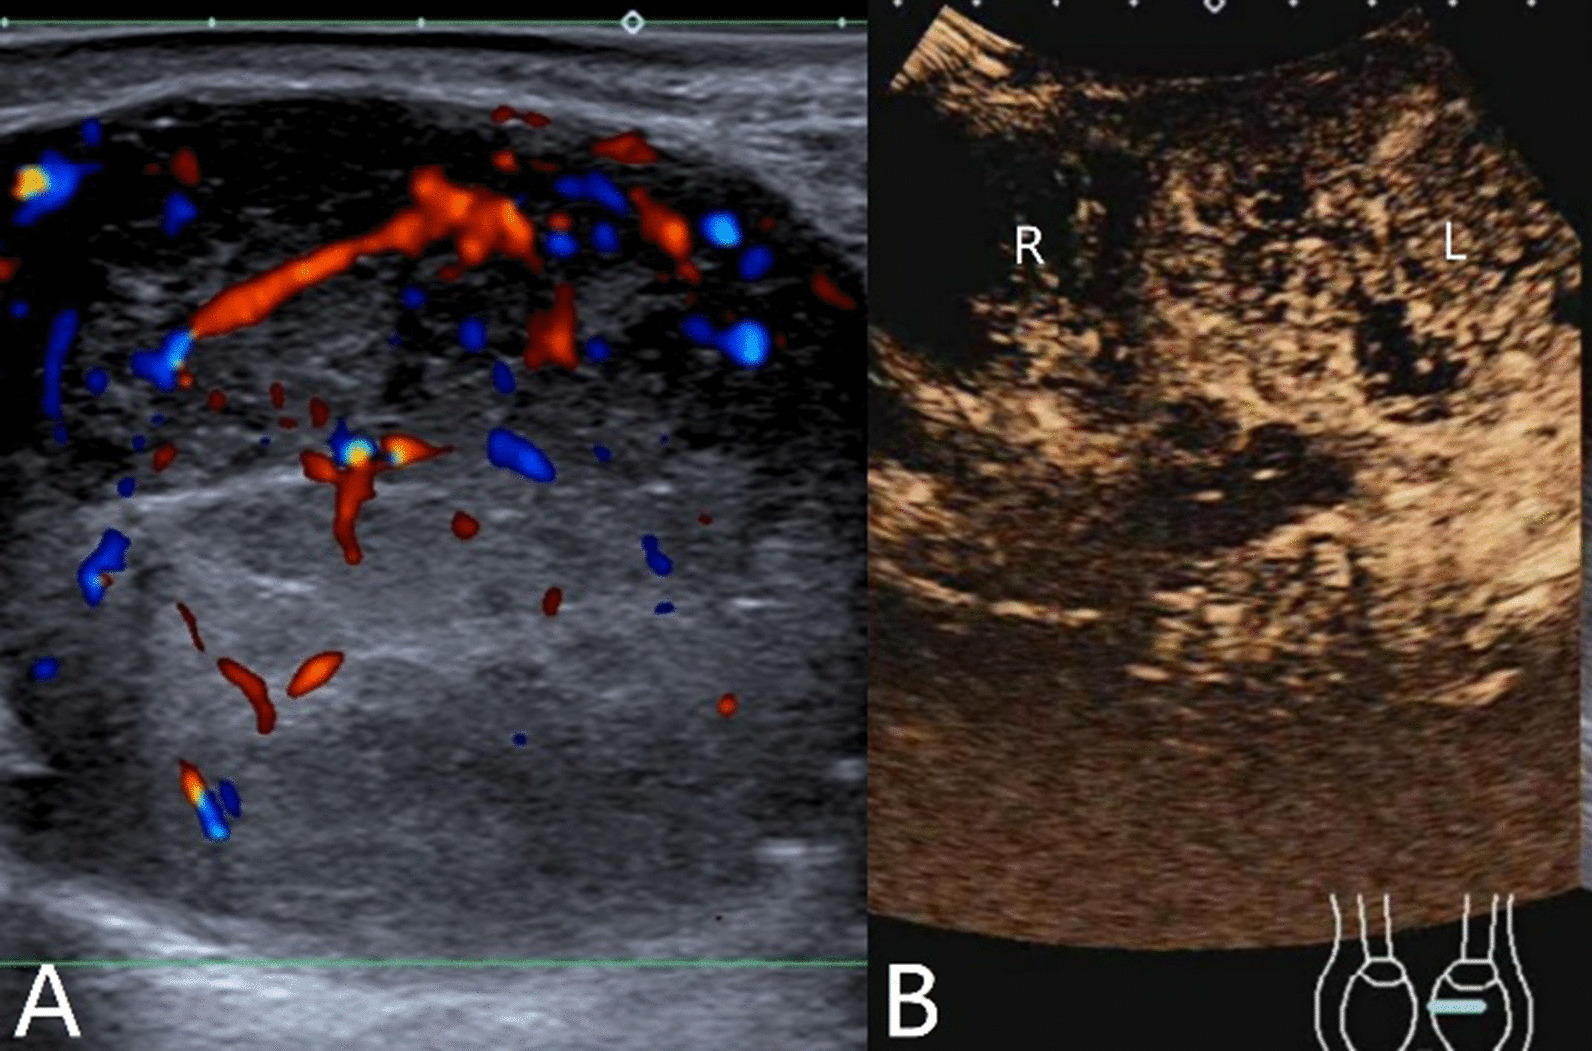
Testicular seminoma. A Conventional ultrasonography showed a round-like hypoecho with a clear border in the left testis and abundant blood flow. B Contrast-enhanced ultrasonography showed non-homogeneous hyperenhancement with necrotic areas
